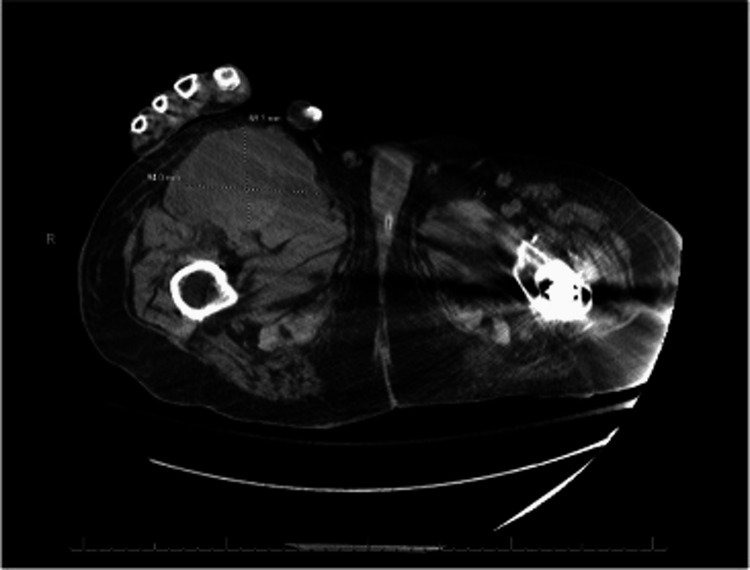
CT abdomen/pelvis without contrast A 4 mm right-sided non-obstructing nephrolith and additional bilateral punctate nephrolithiasis without evidence of hydroureteronephrosis was observed along with a large right anterior thigh fluid collection (6.9 x 1.4 cm) with the focus on internal hemorrhage corresponding to previously seen pseudoaneurysm. A large focal stool burden in the rectum, concerning impaction was obseved. A Foley catheter was in place and urinary bladder mural thickening was suspected.
